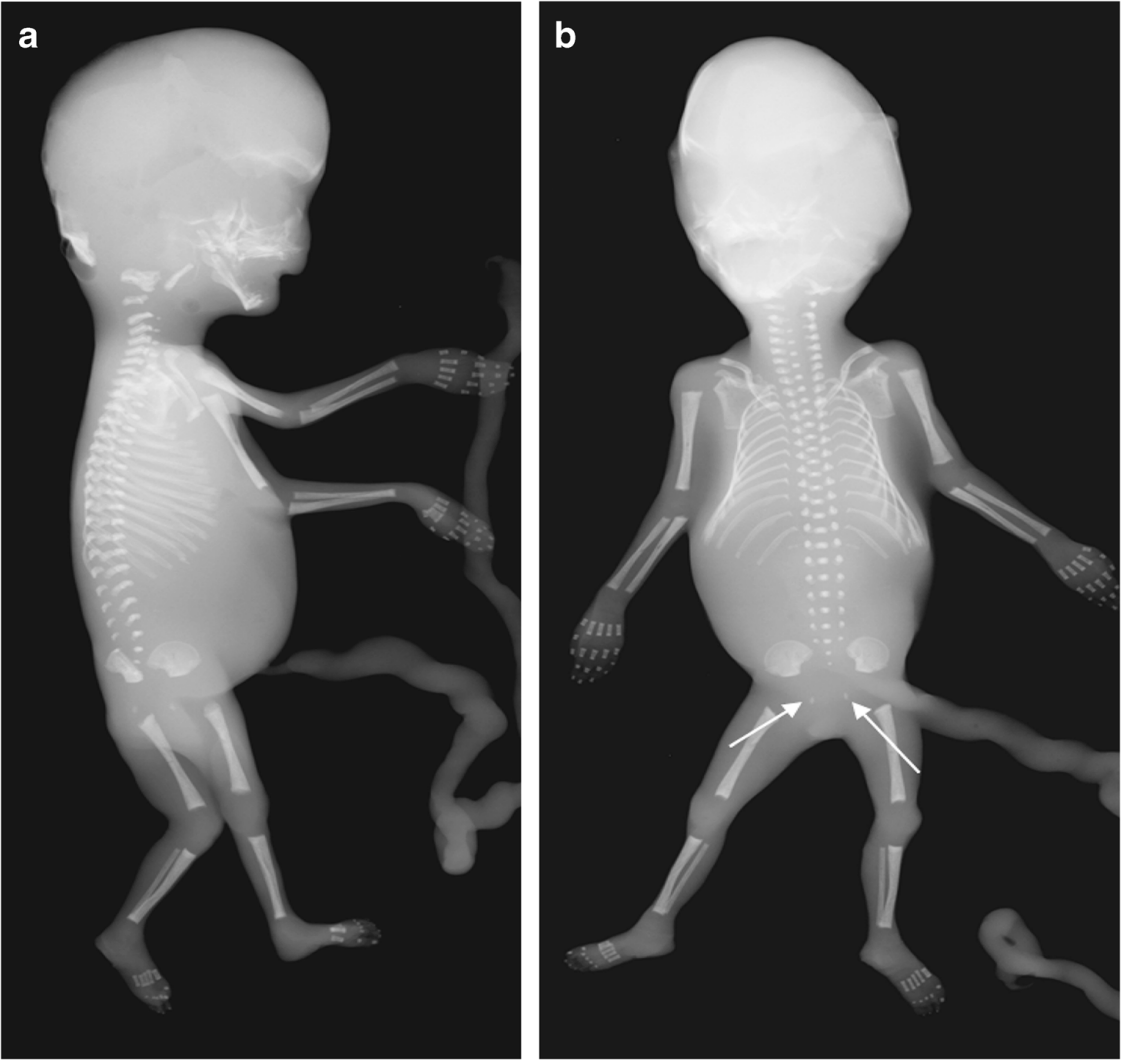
Normal ossification of the fetal skeleton of a male fetus at 15 weeks’ gestation. a,b Radiographs in lateral (a) and anteroposterior (b) projections show early ossification of the ischia at 15 weeks (*arrows*)
